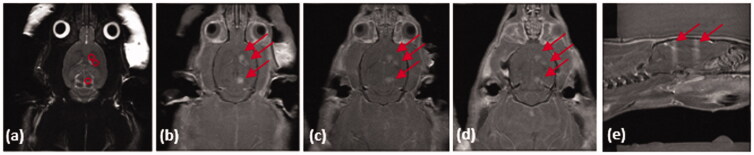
MRIgFUS with AAV2-Sirt3 conjugated MBs causes temporary opening of the BBB in striatum and substantia nigra. (a) The striatum (2 treatment points) and the substantia nigra (1 treatment point) were targeted unilaterally from T2-weighted images (targets are outlined by red circles). Following FUS treatment (US parameters described in material and methods section), the contrast agent gadolinium was given intravenously and (b–d) T1W transverse and (e) saggital images were taken in which the red arrows demonstrate BBB opening. It should be noted that only the sonicated hemishphere demonstrates BBB opening.
